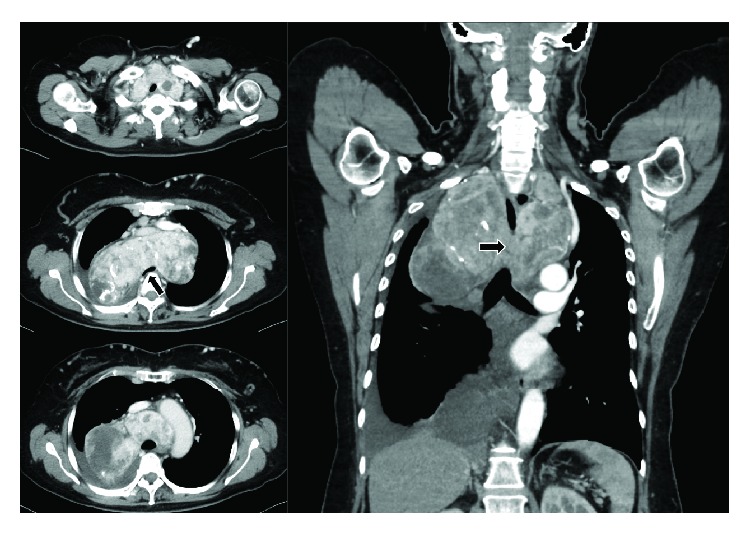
CT findings. CT of the neck and chest showed diffuse swelling of the thyroid gland and a substernal goiter, which extended to both sides of the thorax. The goiter extended to the bifurcation of the trachea on the dorsal side of the superior vena cava, the innominate vein, the aortic arch, and the ventral side of the trachea. It compressed the trachea in the mediastinum, and the lumen of the trachea measured 6 mm in diameter at its narrowest point (arrow). Pleural effusion was also seen in the right thorax.
